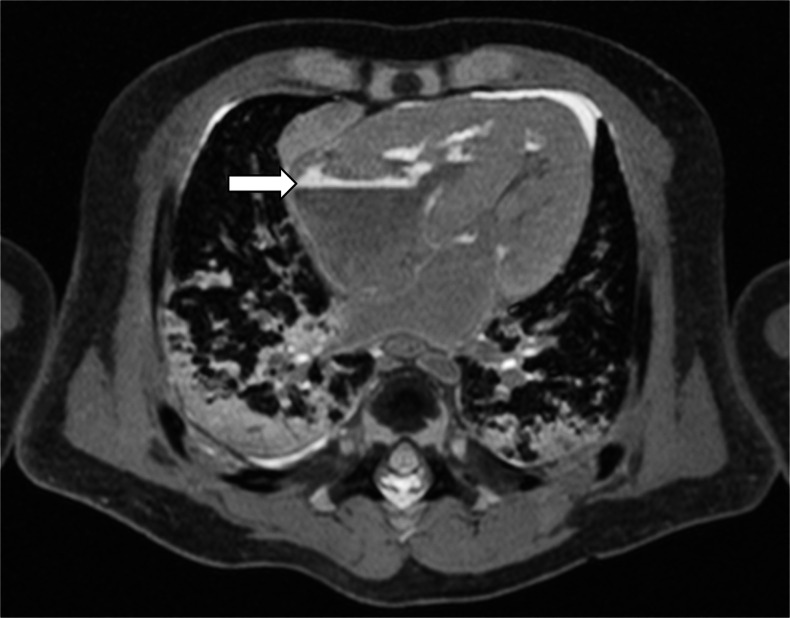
Dependent lung changes. Axial high-resolution T2-weighted postmortem magnetic resonance imaging in a term stillbirth. There are dependent changes in both lungs, which are a normal finding. There is also normal postmortem sedimentation or layering of blood in the heart, shown as a fluid-fluid level (*white arrow*). Note that by imaging earlier in the postmortem interval in this case, fluid redistribution and gas in the heart may not yet have accumulated (compared to Figs. 4 and 5)
